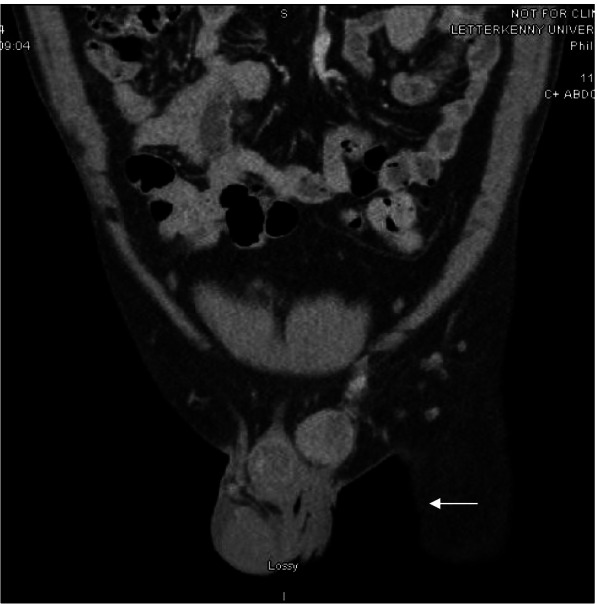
CT showing a mass in the left spermatic cord (arrow)
